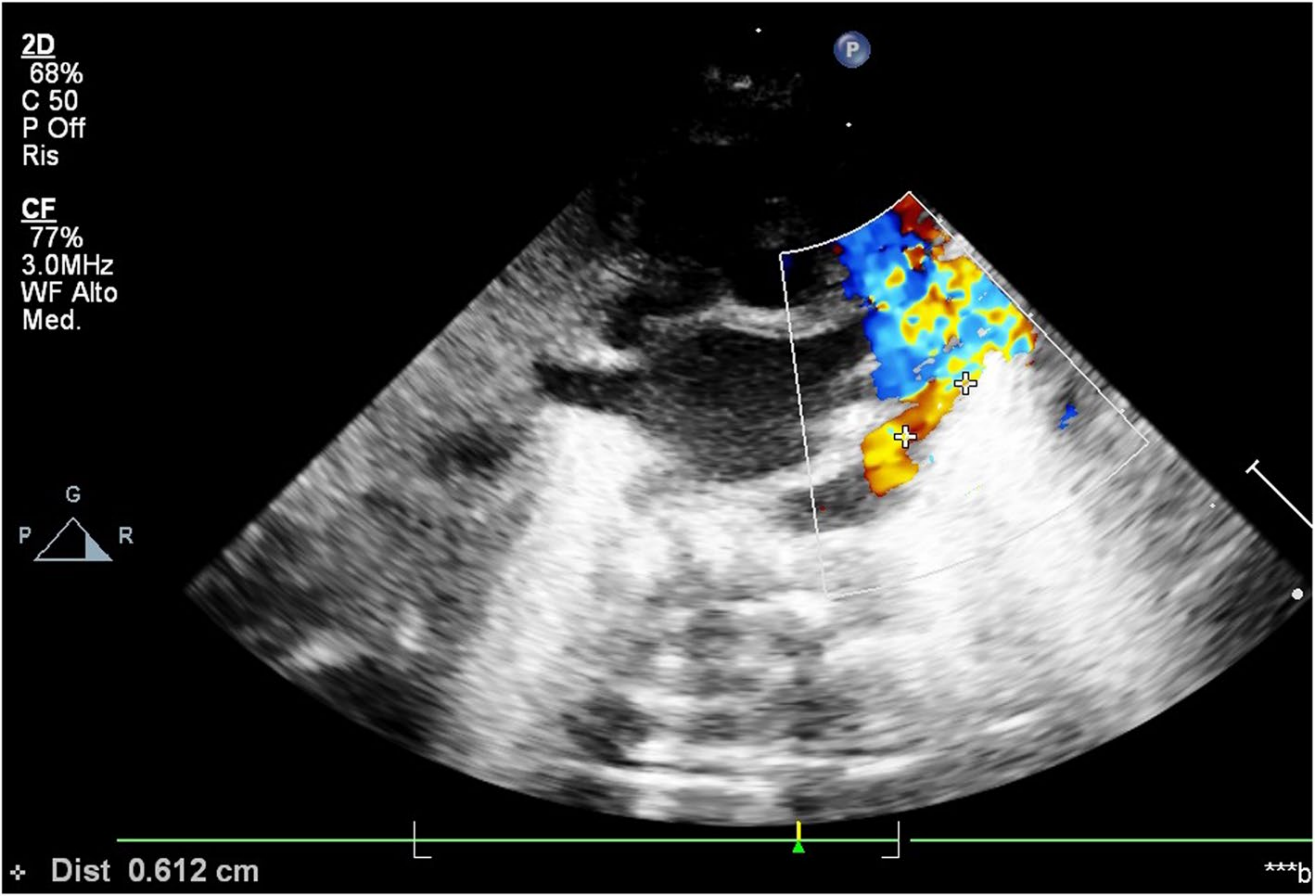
Echocardiographic measurement of the length of the patent duct in a low short axis scan
